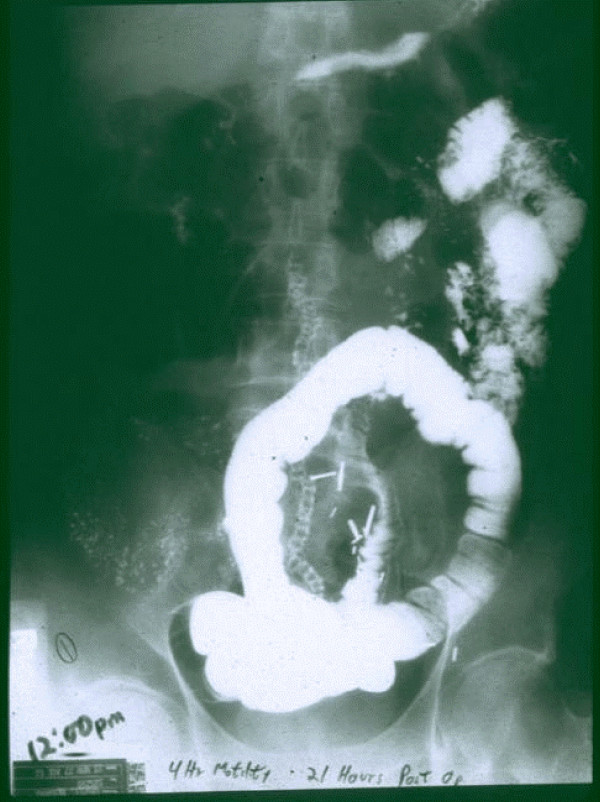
66 y.o. male had sigmoid resection for carcinoma @ 3:00 pm. He was fed immediately for 17 hours via a double lumen, nasoduodenal catheter @ 100 kcal/hr. At 8:00 am the tube was removed, and BaSO4 swallowed. Serial X-rays indicate normal motility. 12:00 noon – 4 hour motility study, 21 hours after surgery.
